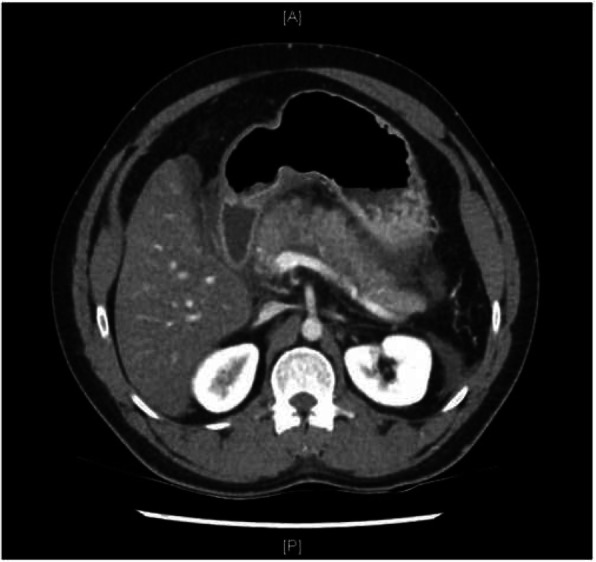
Computed tomography of the abdomen performed on presentation demonstrating findings consistent with uncomplicated acute pancreatitis. Axial view
